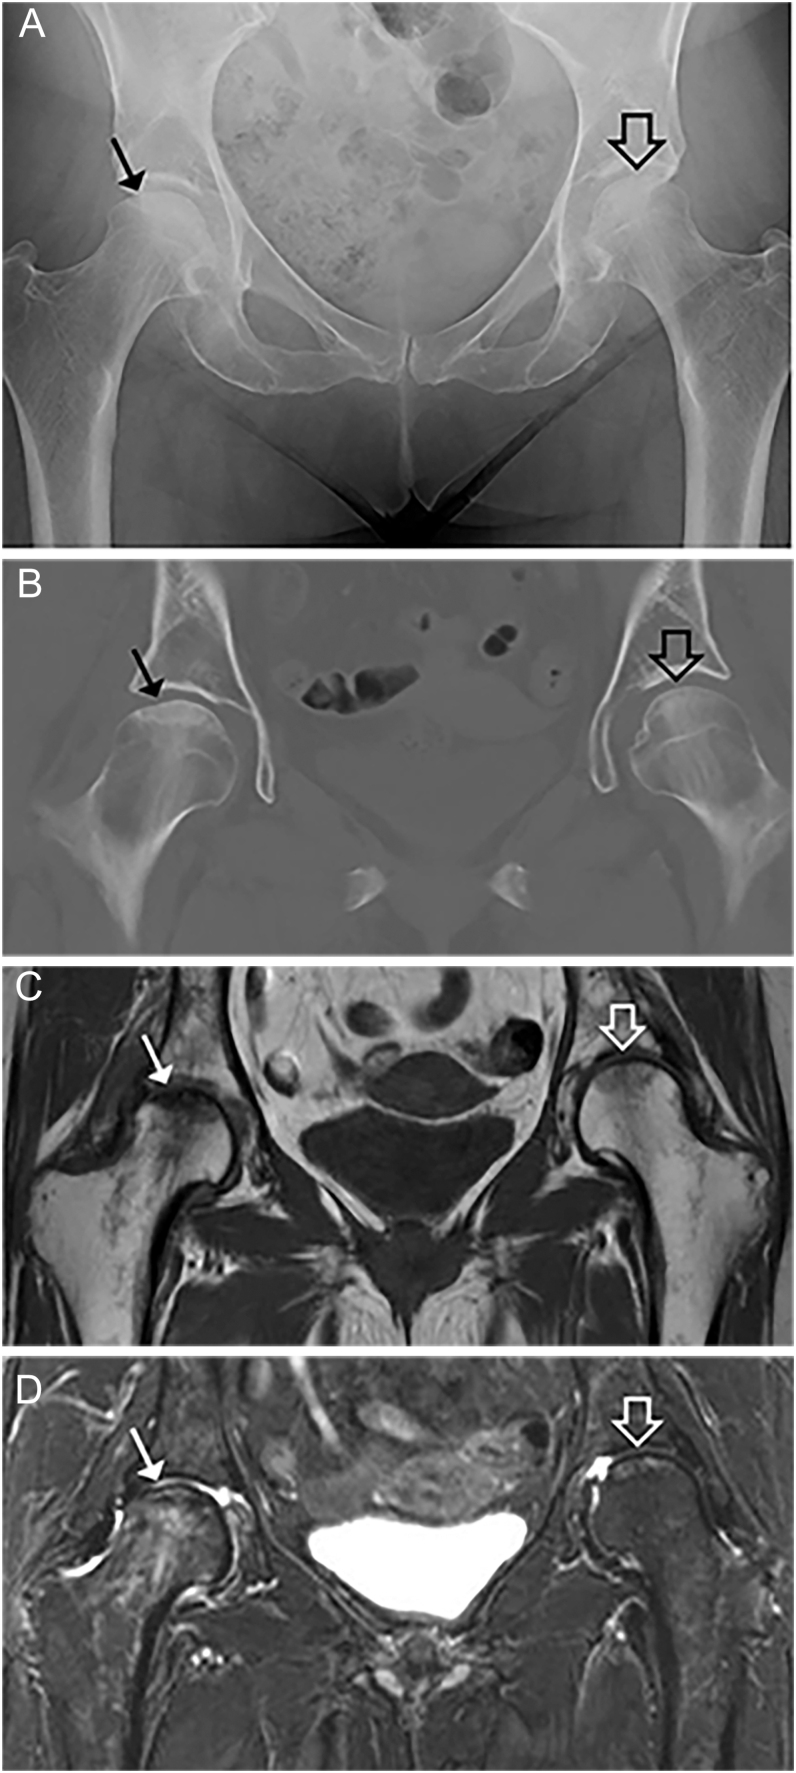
A 59-year-old female with hip pain – bilateral osteonecrosis of the femoral head. AP radiograph of the pelvis (A) and coronal CT of the pelvis (B) demonstrate subchondral sclerosis at the right femoral head with articular surface flattening (arrow), as well as subchondral sclerosis within the left femoral head (open arrow). Coronal T1 (C) and T2 fat-suppressed (D) MR images through the pelvis from MRI acquired one month later demonstrate serpiginous T1 hypointense and heterogeneous T2 signal abnormalities within the femoral heads with subchondral collapse on the right (arrow) but a normal spherical appearance of the left femoral head (open arrow).
